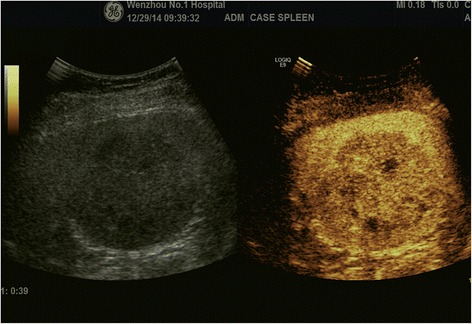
Ultrasound showing splenic hypoechoic lesion appearing with a rounded, clear boundary, heterogeneous enhancement, and centrally irregular necrosis
